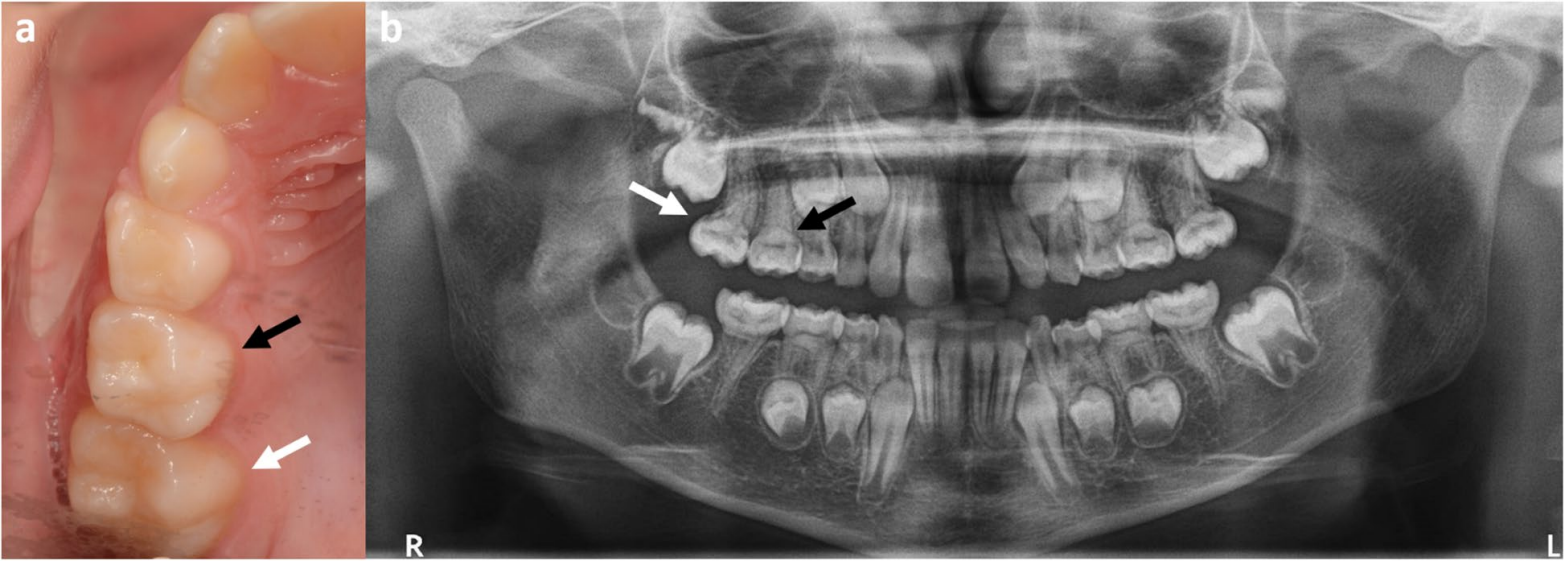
An individual in the mixed dentition with primary second molars and permanent first molars affected by molar-root incisor malformation, a a clinical photograph of the normal clinical crowns of teeth 55 (black arrow) and 16 (white arrow) and b a panoramic radiograph of the same individual with affected teeth 55 (black arrow) and 16 (white arrow) highlighting the tapered, thin roots with a constricted cemento-enamel junction consistent with molar-root incisor malformation
